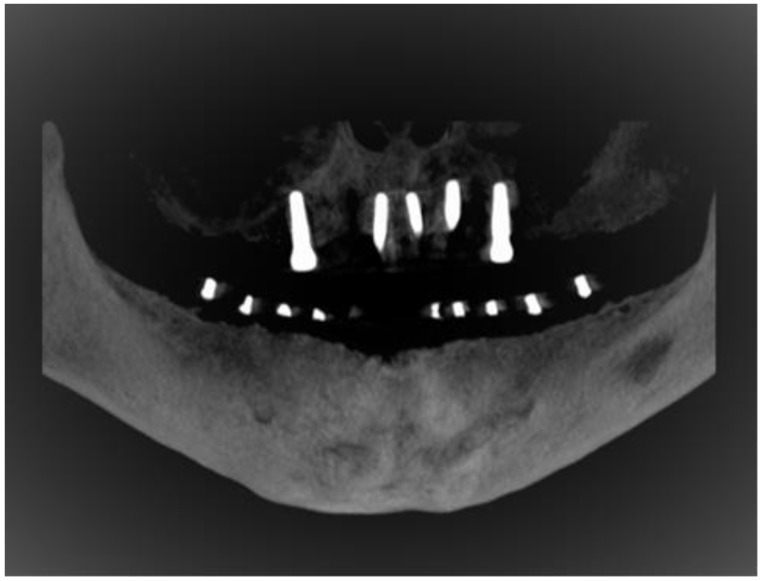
Reformatted panoramic view on CBCT for edentulous mandibular ridge with customized stent.
